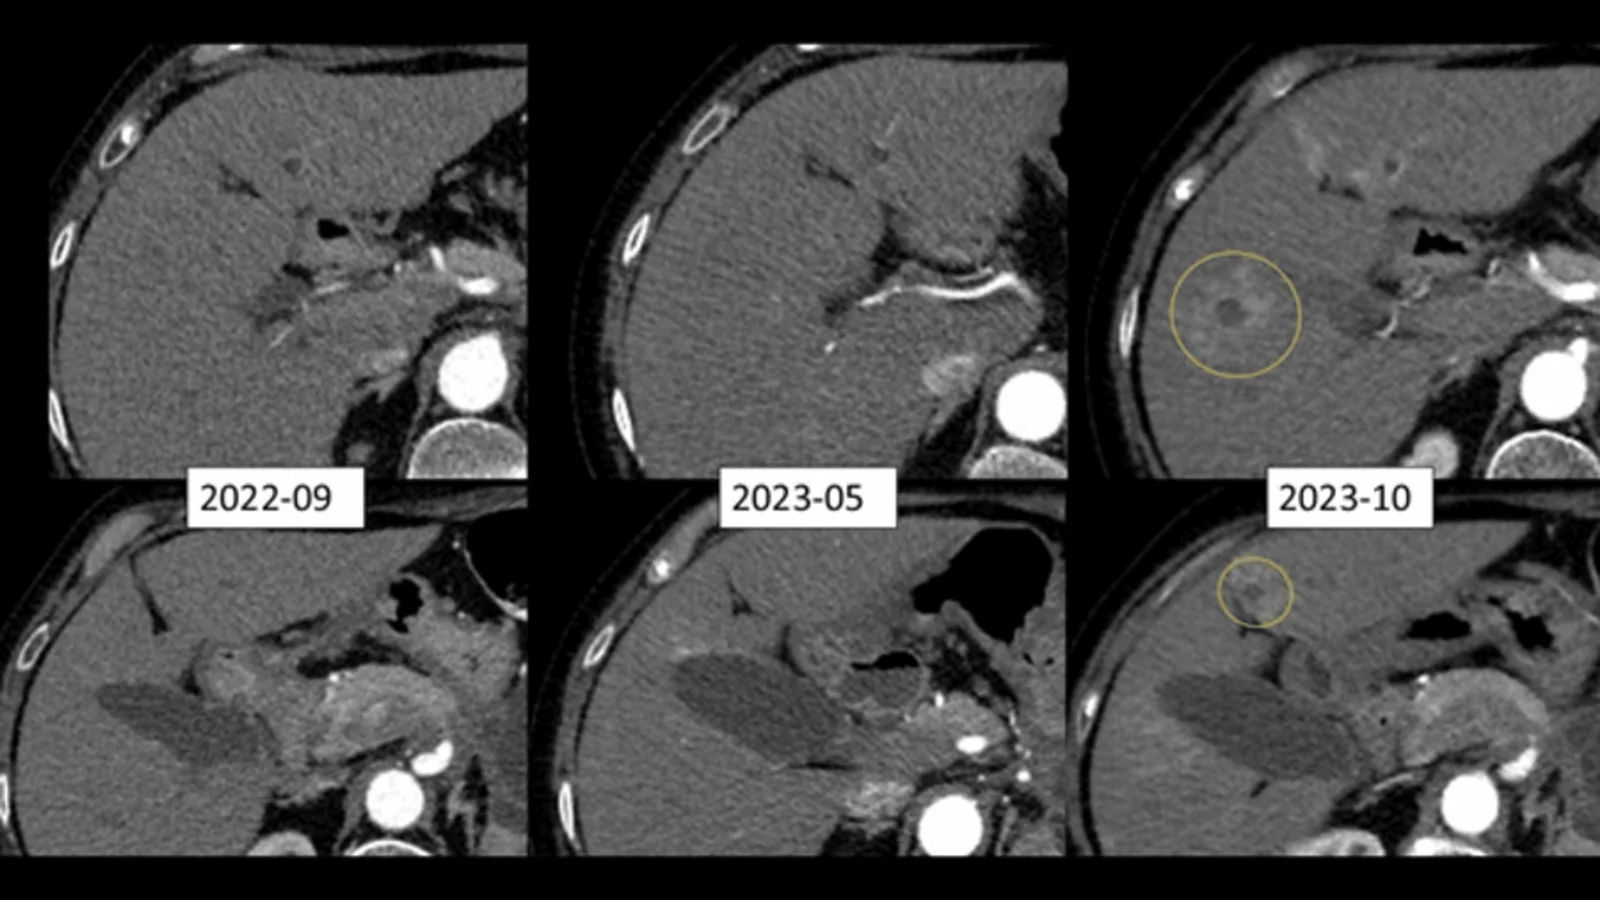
Serial CT imaging of the liver showing the development of metastatic lesions The yellow circle shows a metastatic lesion in the liver. CT, computed tomography.
